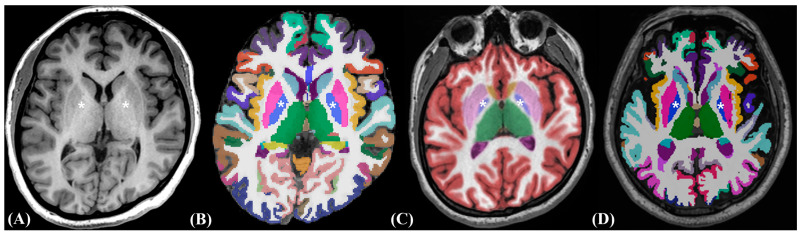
Representative color-coded axial MR images at the level of the basal ganglia. An axial T1-weighted image (A) is shown at the basal ganglia level with color-coded images of the FS, NQ, and HAD. In these representative images, the pallidum appears smaller in NQ (C) than in FS (B) or HAD (D). The pallidum is indicated with asterisks. FS, FreeSurfer; HAD, Heuron AD; NQ, NeuroQuant.
